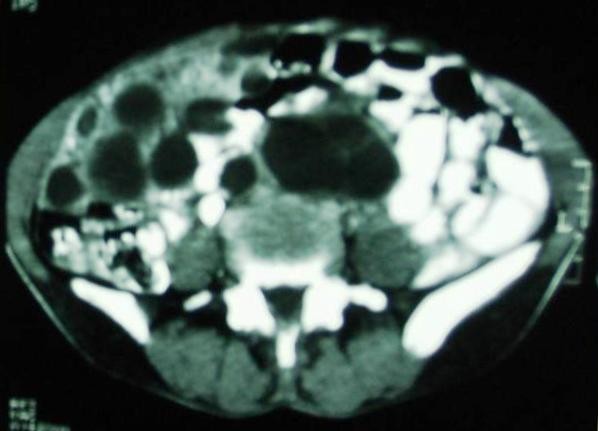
CT scan showing multiple thin walled cysts.
